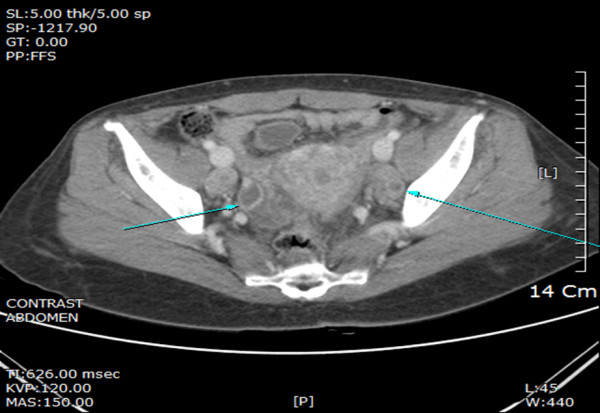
Abdominopelvic computed tomography (CT). Both ovaries appear normal, but the right ovary is slightly enlarged.
